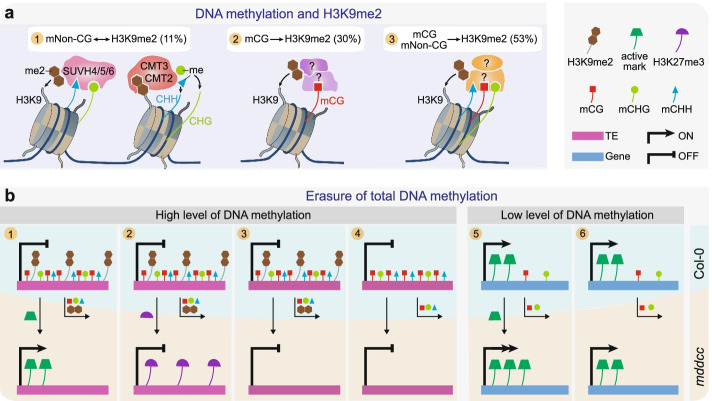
Function of DNA methylation in histone modification and transcription landscapes. a Distinct models of DNA methylation in regulating H3K9me2. (1) A feedback loop between non-CG methylation and H3K9me2: non-CG methylation recruits the H3K9 methyltransferases SUVH4, SUVH5 and SUVH6, and in turn, H3K9me2 facilitates CMT3 and CMT2 function to methylate CHG and CHH; (2 and 3) most of H3K9me2 is regulated by CG methylation (2) or by both CG and non-CG methylation (3), through yet unclear mechanisms. mCG, methylated CG; mNon-CG, methylated non-CG including methylated CHG and CHH. b Representative models for the impacts of DNA methylation on histone modifications and TE/gene transcription. Descriptions are in main text
